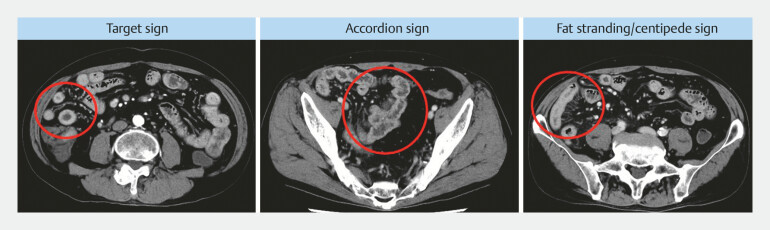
Findings of small bowel inflammation on CT.
